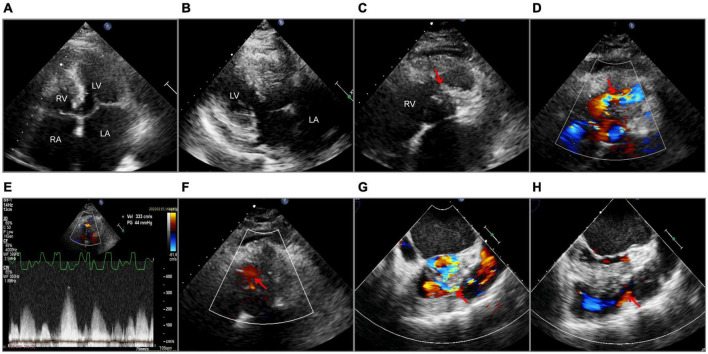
Echocardiography. Transthoracic echocardiography (A–F). (A) A 2-D apical four-chamber view with enlarged atria. (B) Left ventricular hypertrophy and interventricular septal hypertrophy. (C) Muscular septation within the right ventricle (the red arrow). (D) Flow acceleration showed by Color Doppler at the right ventricular outflow tract (the red arrow). (E) The systolic pressure gradient of the right ventricular outflow tract. (F) Ventricular septal defect (the red arrow) detected by Color Doppler. Transesophageal echocardiography (G,H). Color Doppler flow of the right ventricular outflow tract before (G) and after operation (H), as shown by the red arrows. LA, left atrium; RA, right atrium; LV, left ventricle; RV, right ventricle; Vel, velocity; PG, pressure gradient.
